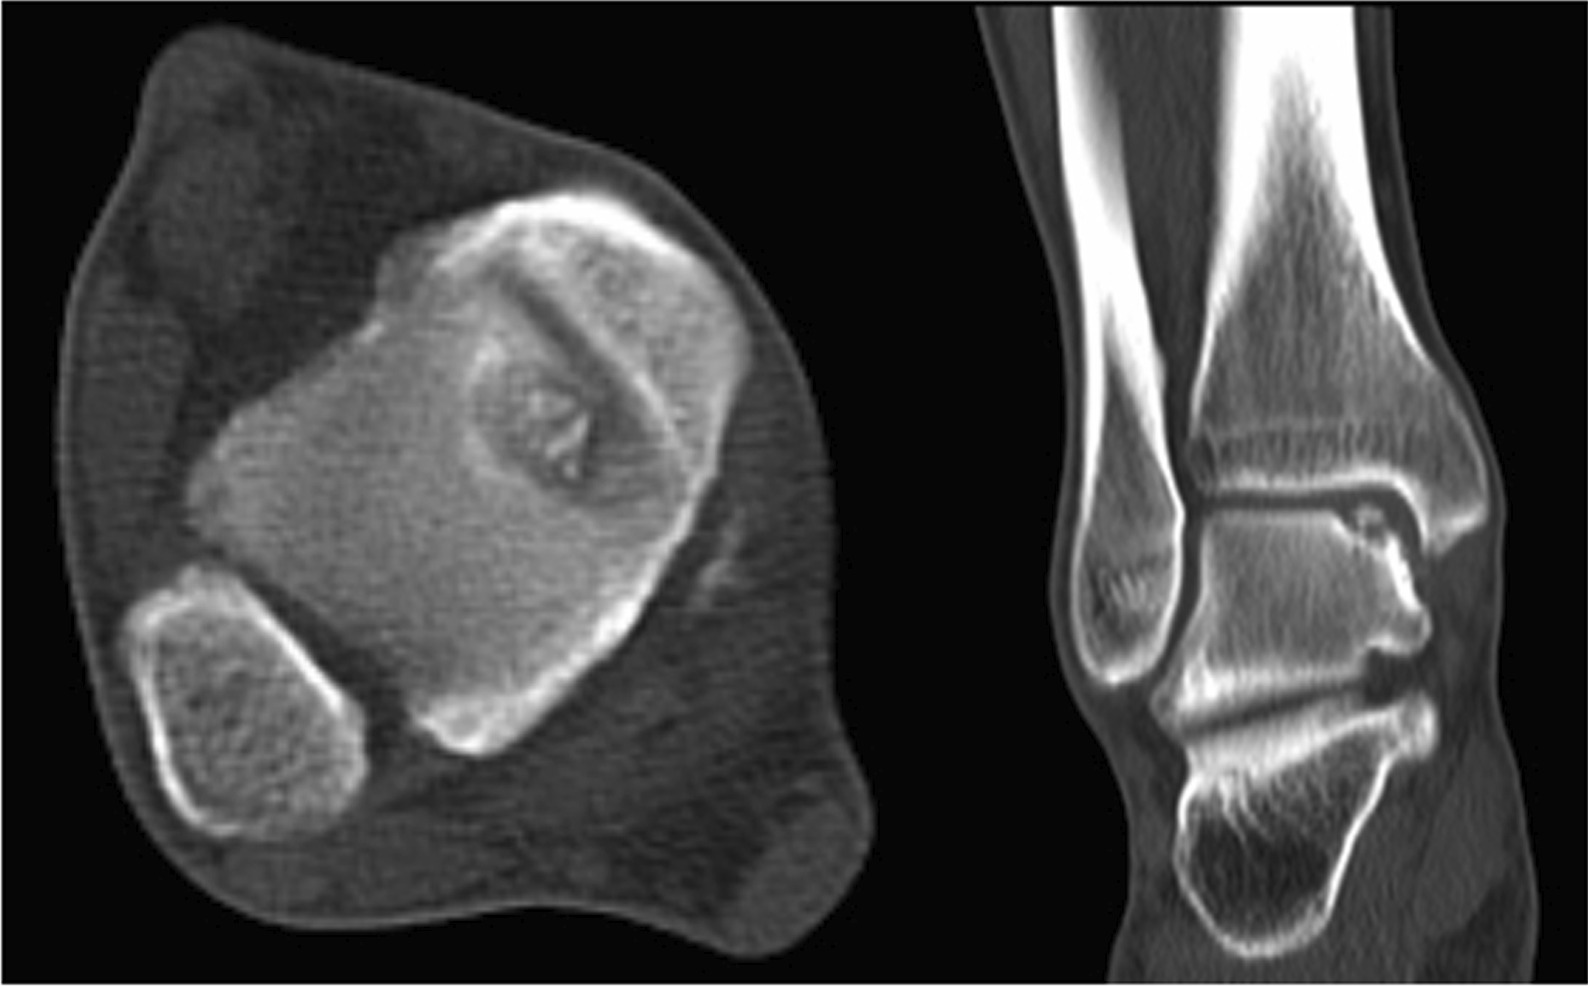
Preoperative CT shows medial talus necrosis
